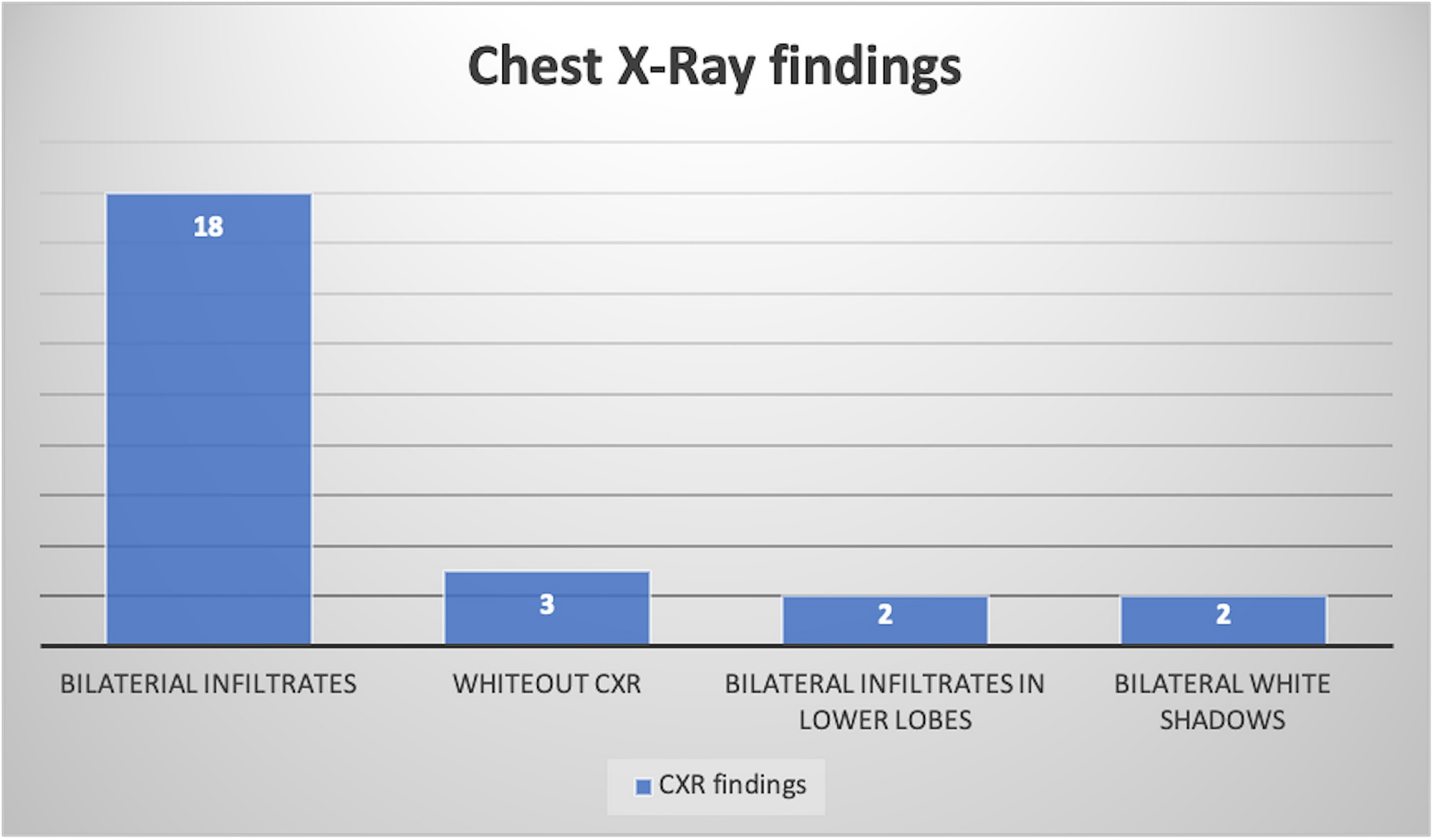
CXR findings observed among the study cohort. CXR: chest X-ray
